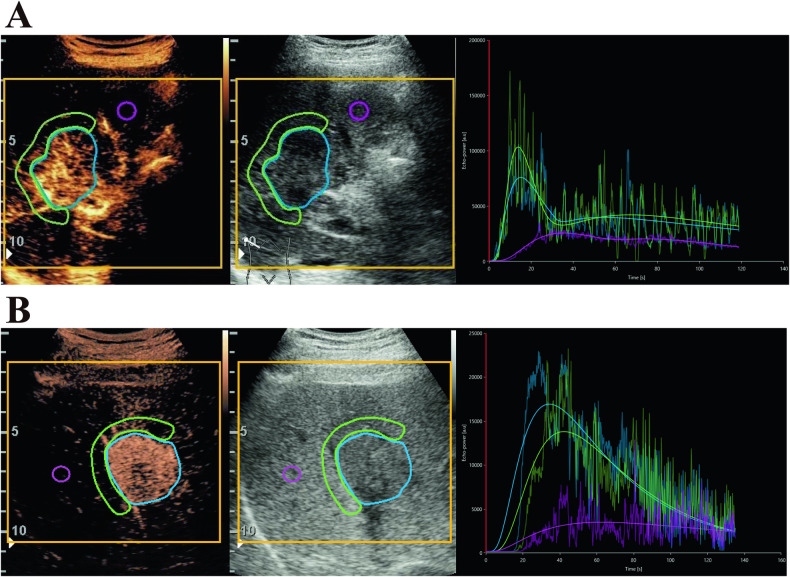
Dynamic contrast-enhanced ultrasound (DCE-US) perfusion analysis using the VueBox®. Blue regions of interest (ROIs) are placed around the whole intrahepatic cholangiocarcinoma (ICC) lesion, green ones at the margin of the ICC lesion, and pink ones in the surrounding liver parenchyma accordingly. Time-intensity curves (TICs) of the contrast agent in the ROIs are generated. A LD-type ICC, B SD-type ICC
